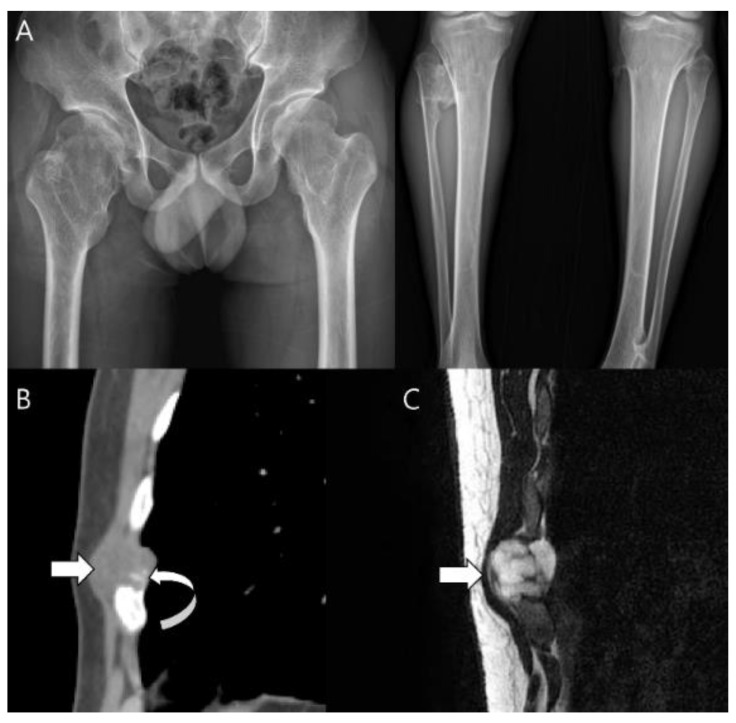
Secondary peripheral chondrosarcoma of the rib in a 34-year-old man. (A) Hip and tibial plain radiographs reveal underlying multiple exostoses. (B) Sagittal CT scan shows a lobulated mass with soft-tissue density (arrow) arising from the rib containing matrix mineralization (curved arrow), suggestive of a cartilage cap of sessile osteochondroma. (C) Sagittal T2-weighted image shows a mass of 2.3 cm in thickness with high signal intensity (arrow).
